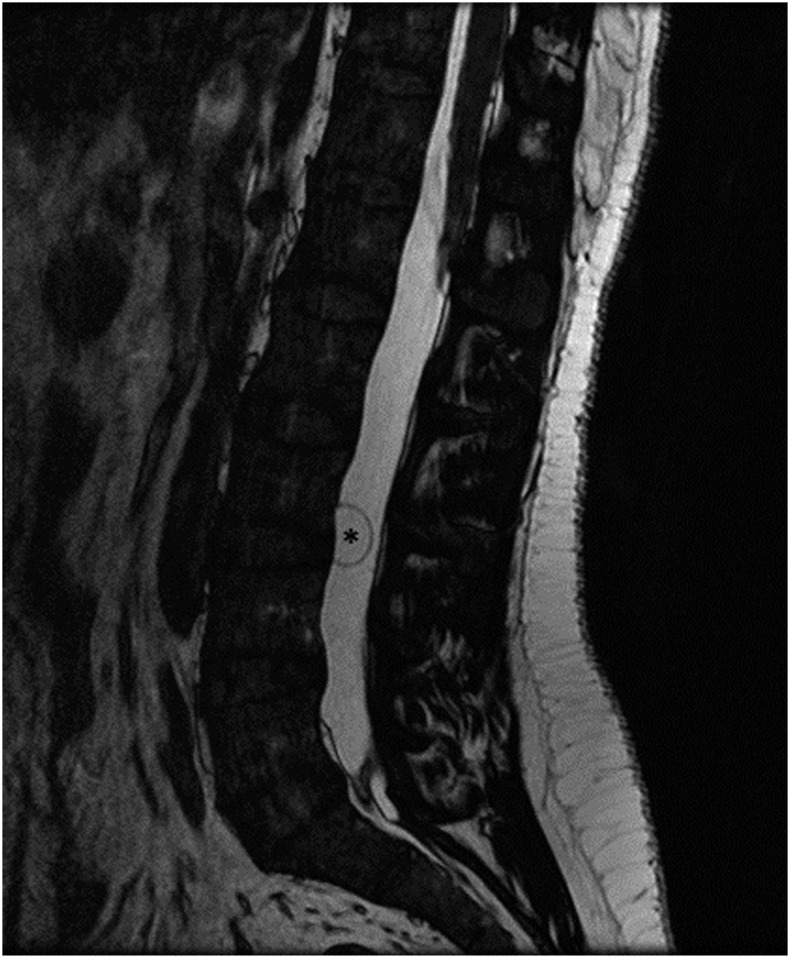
Spinal MRI: volumetric T2-weighted imaging in the sagittal plane shows a large intradural extramedullary cyst behind the L3 vertebral body (asterisk).
